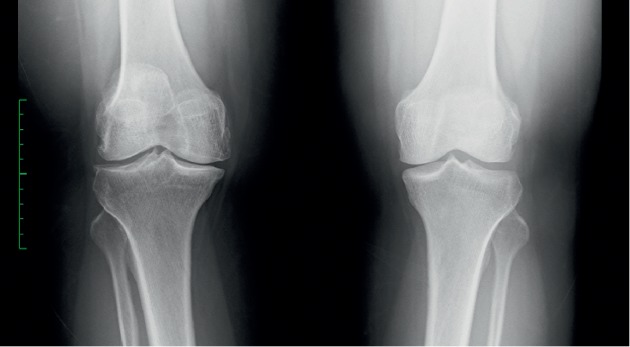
X-ray examination in case 2.
